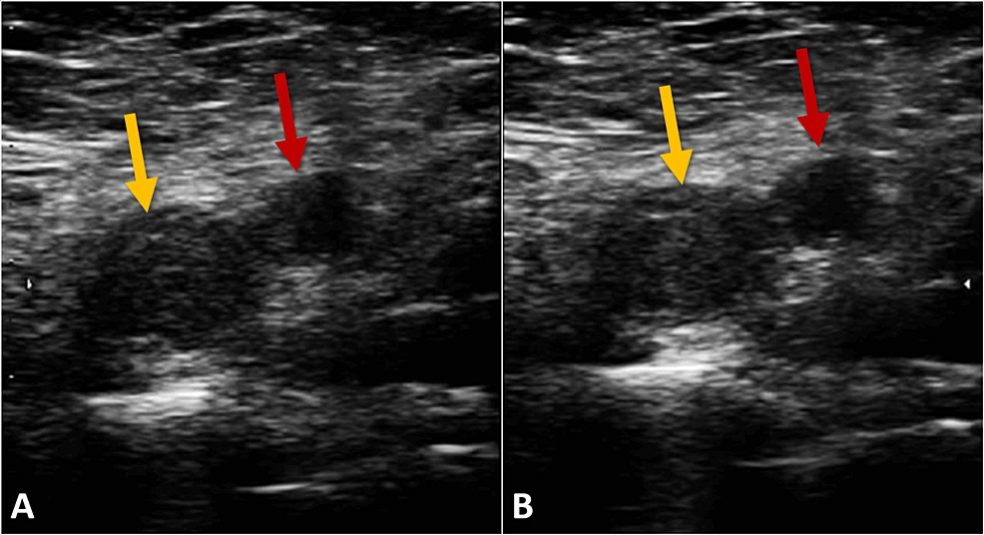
Color Doppler ultrasound of the femoral vein This color Doppler ultrasound image showcases the femoral vein (yellow arrow) and the femoral artery (red arrow), highlighting their normal anatomical positions without compression (A). The inability of the femoral vein to collapse under applied compression (B) indicates a critical diagnostic sign of deep vein thrombosis.
